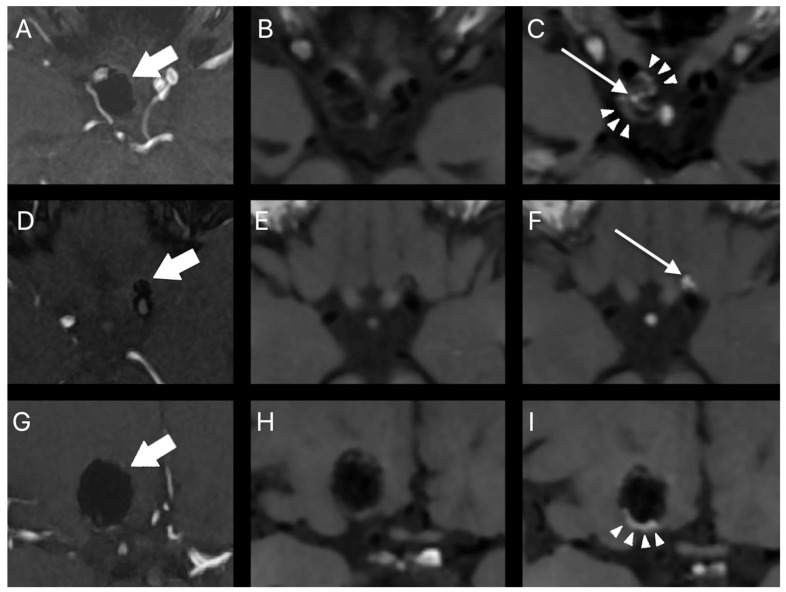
Representative cases of post-procedural aneurysm contrast enhancement on HR-VW MRI. Follow-up TOF MRA (A,D,G) and corresponding precontrast (B,E,H) and postcontrast (C,F,I) HR-VR MRI. Right internal carotid artery aneurysm treated with stent-assisted coiling (thick arrow) (A–C). There is no residual flow in the aneurysm cavity. Postcontrast HR-VW MRI shows contrast enhancement at the aneurysm periphery (arrowheads) as well as in the aneurysm cavity (thin arrow). Left internal carotid artery aneurysm treated with stent-assisted coiling (thick arrow) (D–F). Follow-up TOF MRA shows complete aneurysm occlusion. Postcontrast HR-VW MRI demonstrates intense contrast enhancement in the aneurysm cavity (thin arrow) with no contrast enhancement at the aneurysm periphery. Right internal carotid artery aneurysm treated with stent-assisted coiling (thick arrow) (G–I). The aneurysm is completely occluded. There is a thin rim of contrast enhancement at the aneurysm periphery (arrowheads) on postcontrast HR-VW MRI. No contrast enhancement in the aneurysm cavity is visible.
